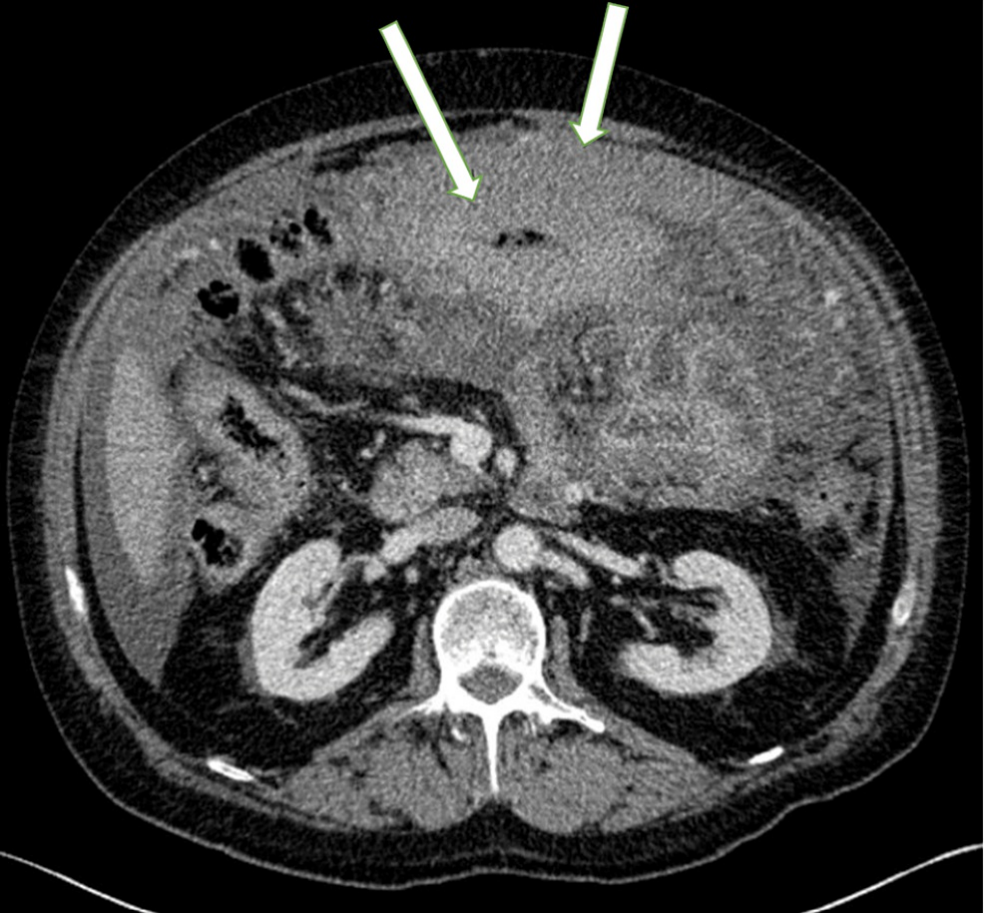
CT scan of the distal transverse colon CT scan findings show short segment circumferential wall thickening at the distal transverse colon which suggests luminal narrowing (see arrows).
